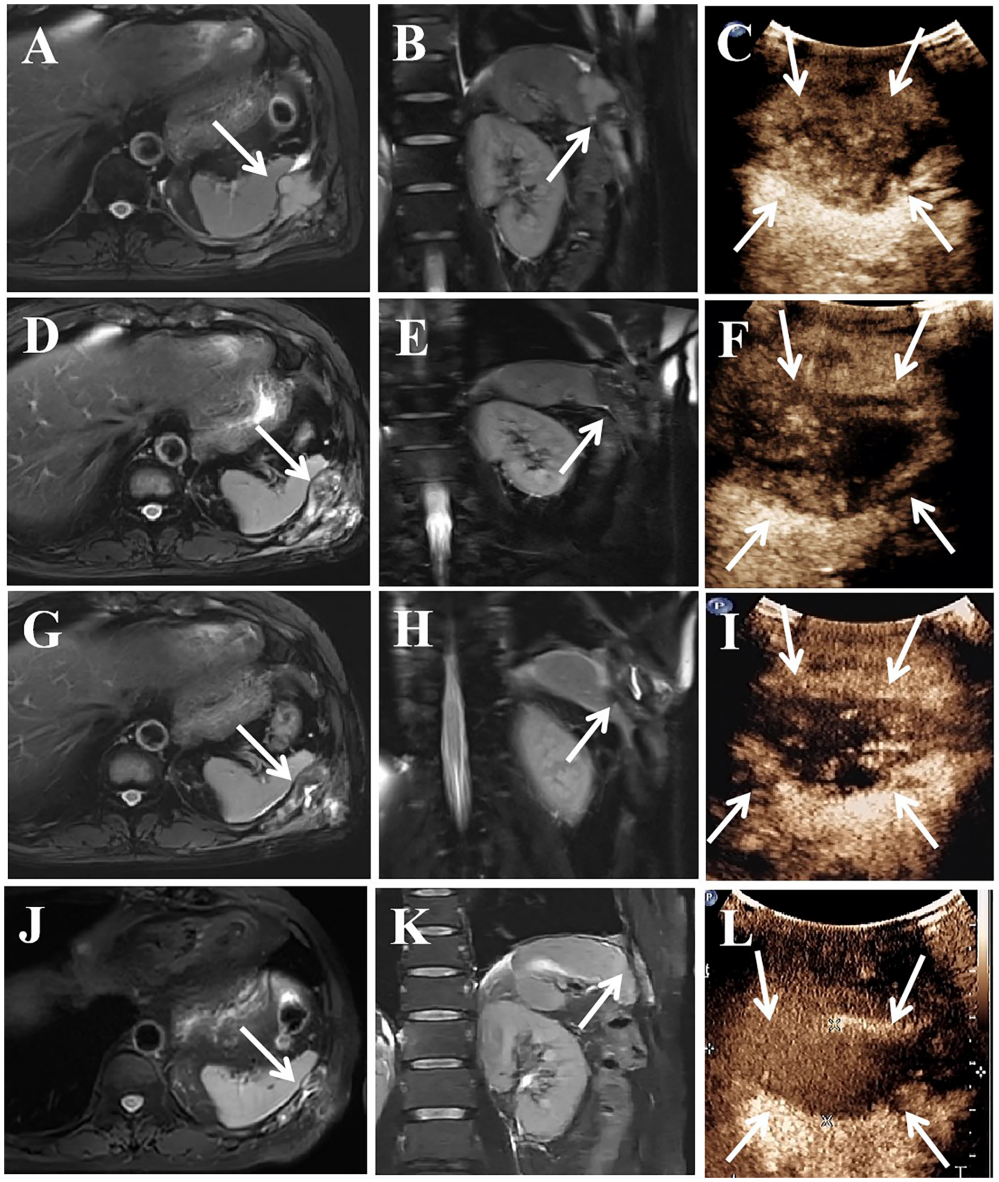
A 58-year-old man treated GIST metastases in the chest wall with MWA. (A–C) In January 2022, preoperative MRI showed a mass of 5.6×3.2 cm in the rib, and CEUS showed heterogeneous enhancement. (D–F) In February 2022, the tumor size was similar to before, CEUS showed partial enhanced area. (G–I) In April 2022, the tumor size was 5.3×2.4cm, and CEUS showed enhanced area. (J–L) 17 months after the second ablation, the tumor size was 4.7×1.6 cm, and CEUS showed no enhancement. MRI, magnetic resonance imaging; CEUS, contrast-enhanced ultrasound; the white arrow, lesion in the rib.
